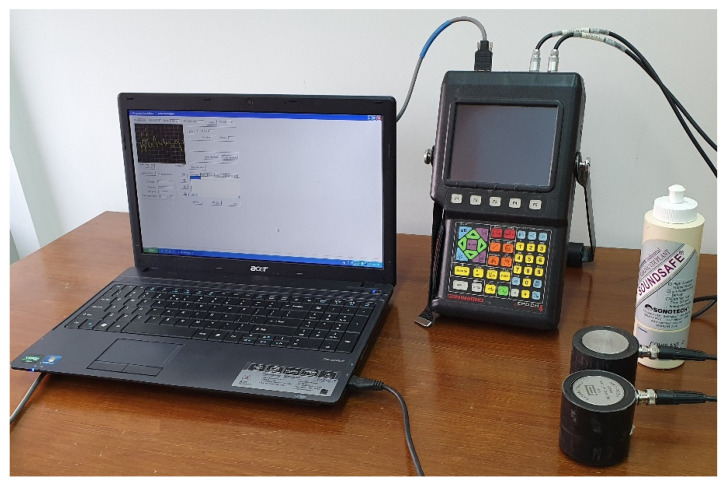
The ultrasonic experimental setup used in the study–ultrasonic defectoscope Epoch4, piezoelectric transducers of 100 kHz central frequency and coupling gel (Panametrics NDT-Olympus NDT, Shinjuku, Tokyo, Japan).
